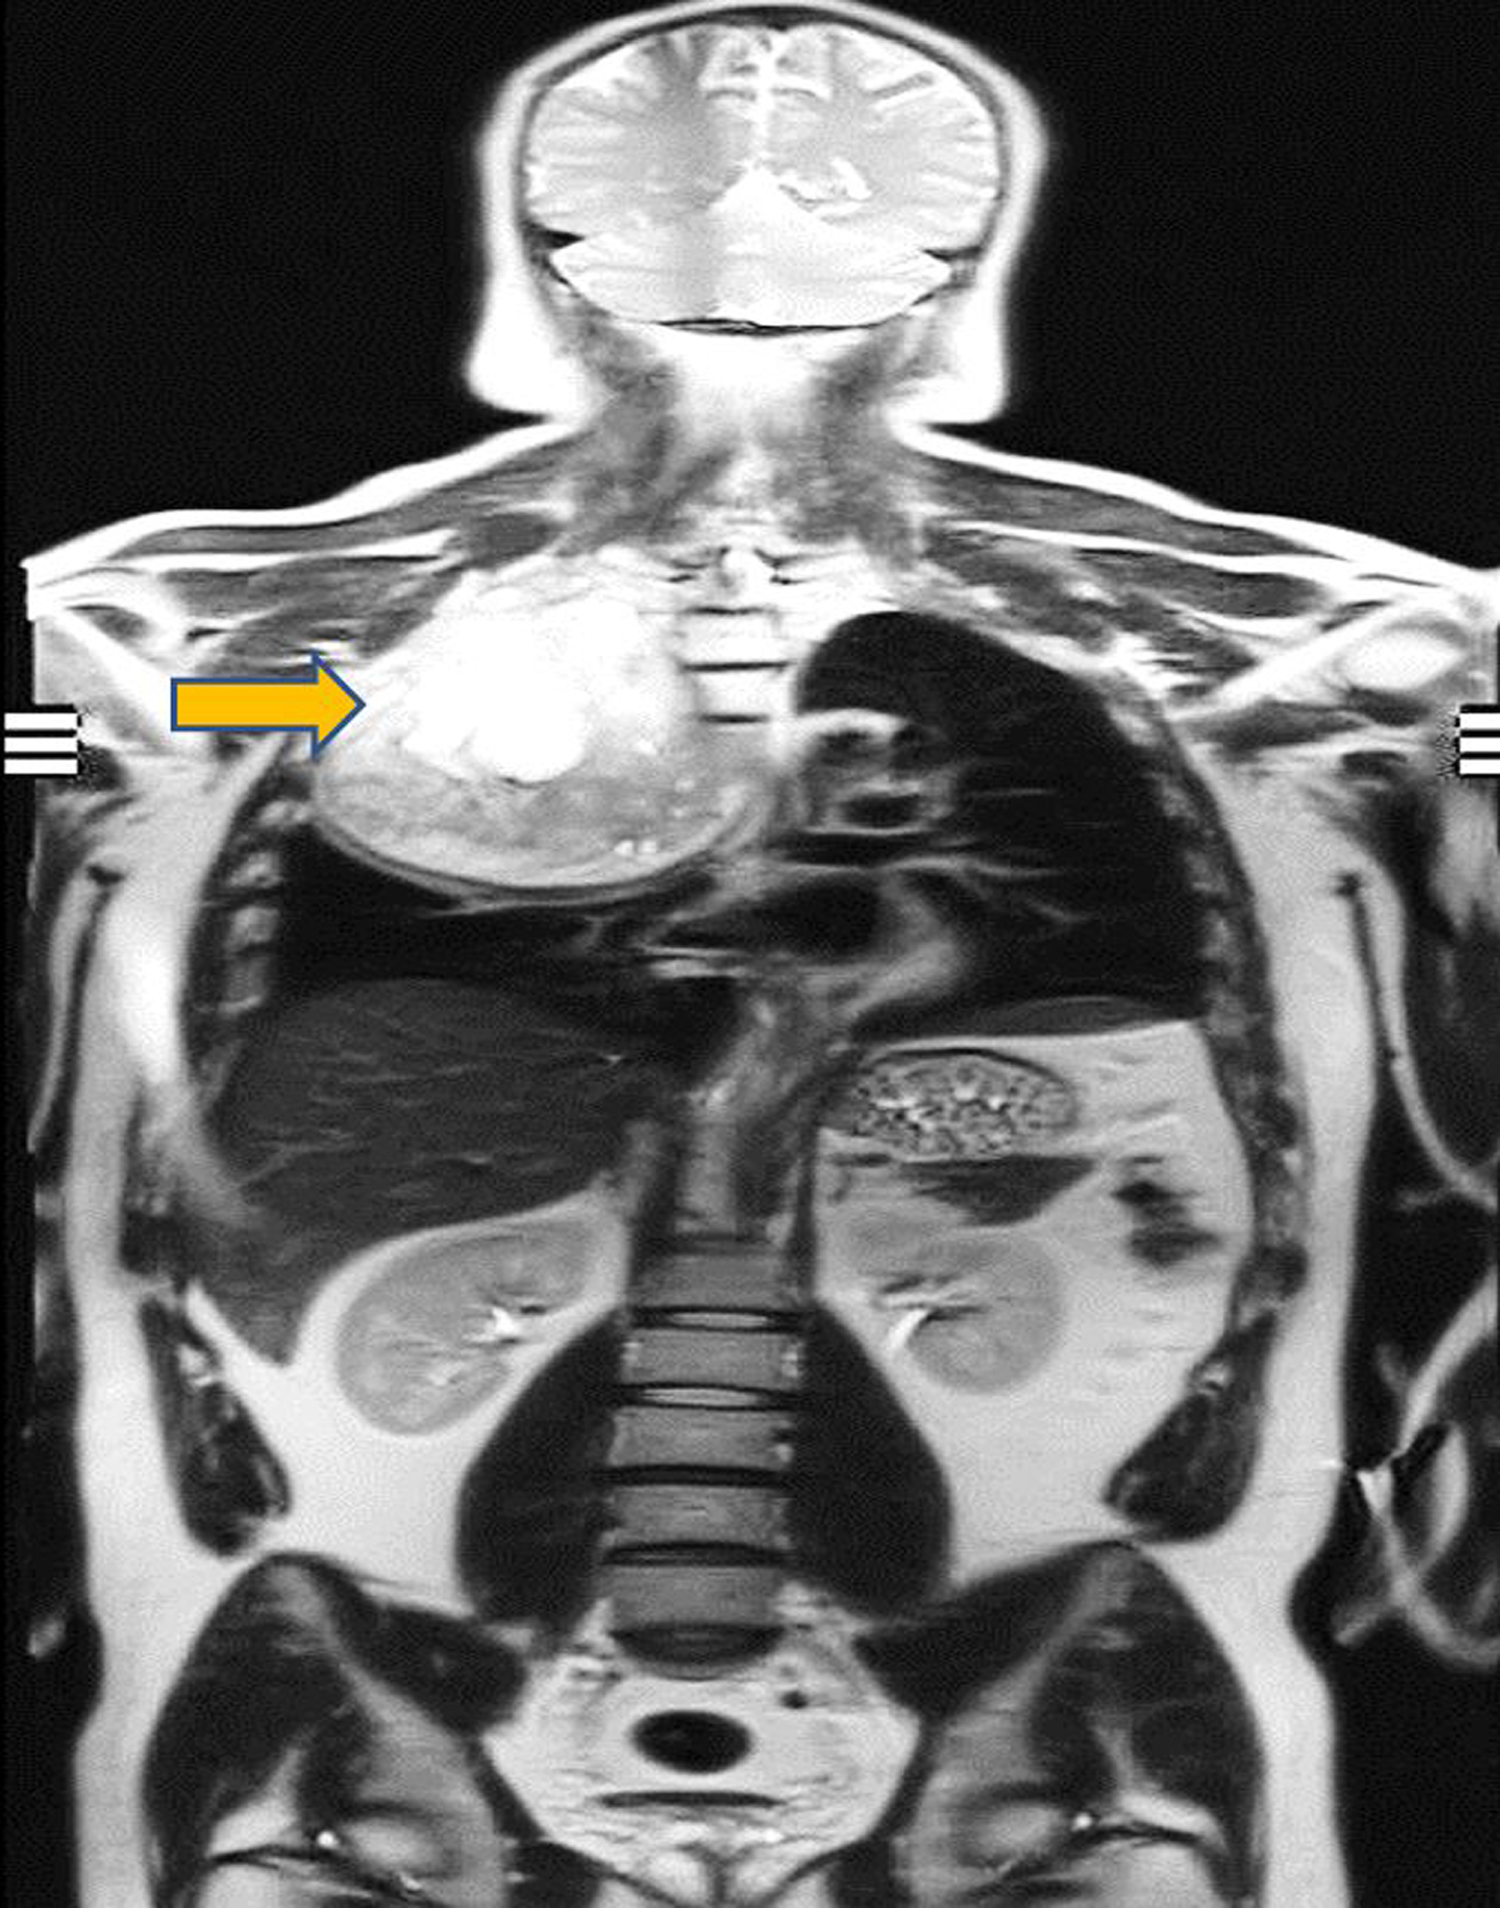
Preoperative Chest MRI image: Heterogeneously enhancing mass (arrows) in the right thoracic inlets and lung apex (13.4 cm × 12.3 cm).
